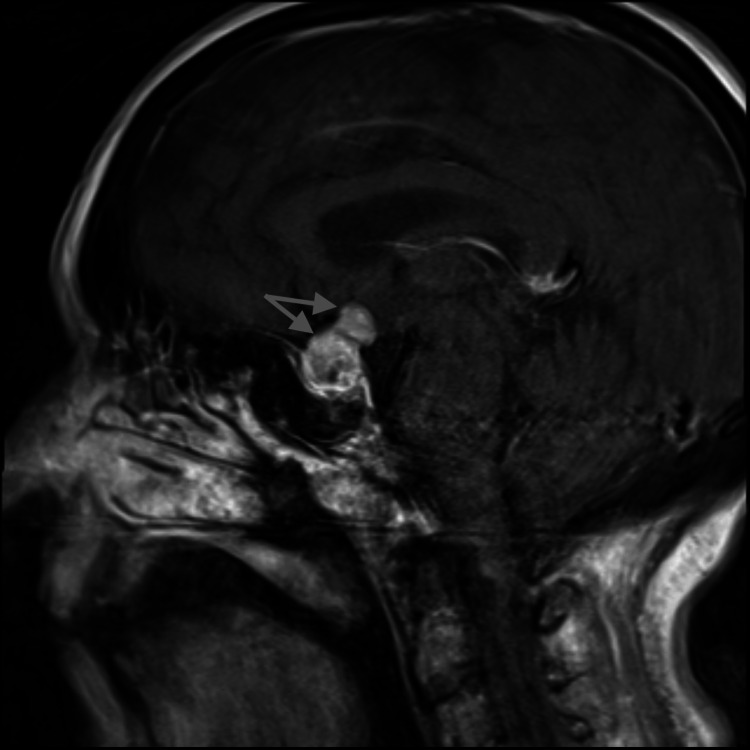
T1 post contrast brain MRI with pituitary protocol (sagittal image) Image showing a dumbbell lesion in the pituitary consistent with metastatic disease to the pituitary Gray arrows: pituitary mass
